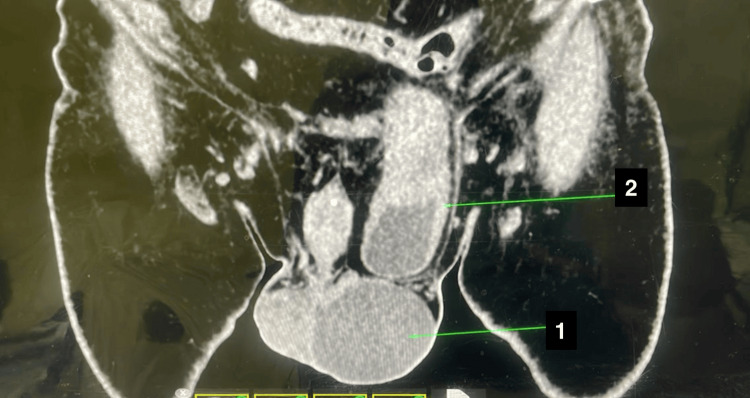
CT reports showing a hydrocele and herniated urinary bladder in coronal view; arrow 1 indicates a hydrocele sac; arrow 2 indicates an irreducible urinary bladder
